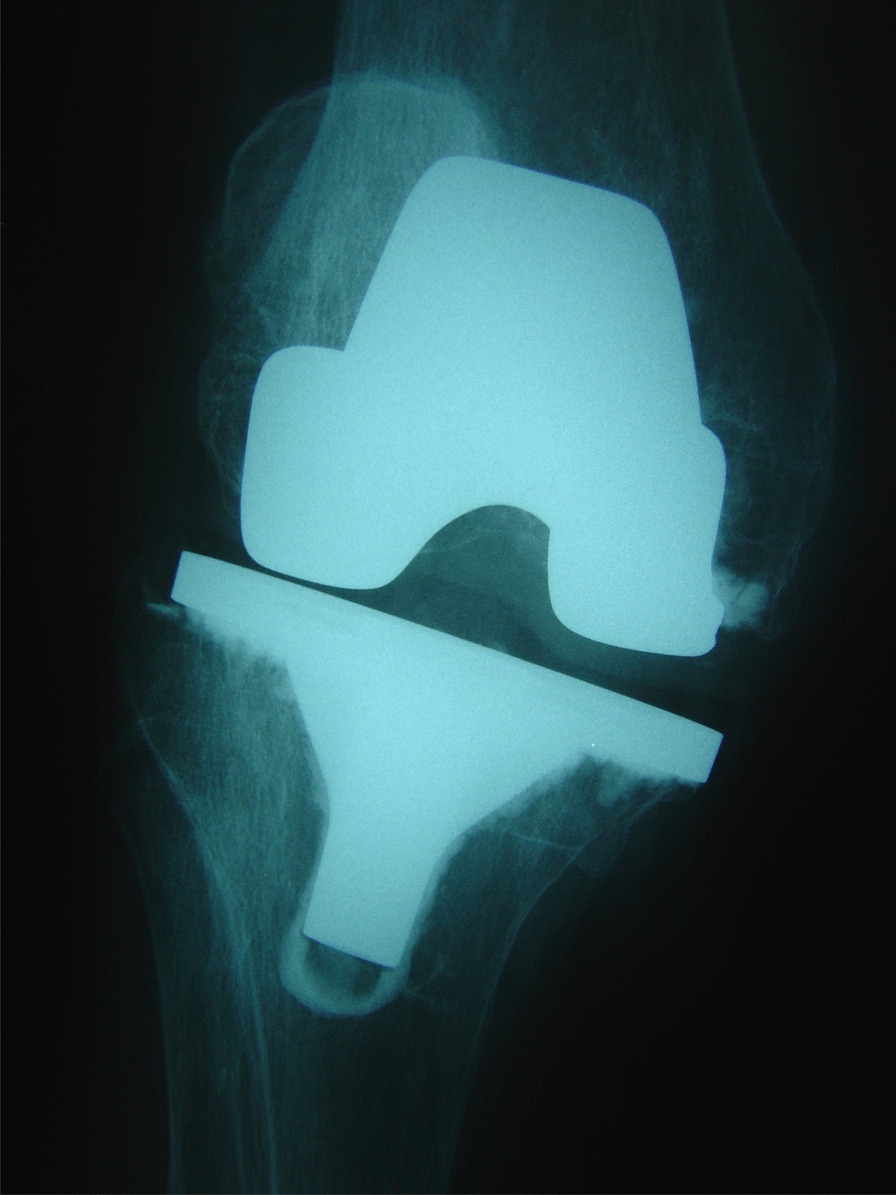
This AP radiograph demonstrates significant laxity to valgus stress due to malalignment of the tibial component which ultimately required revision
